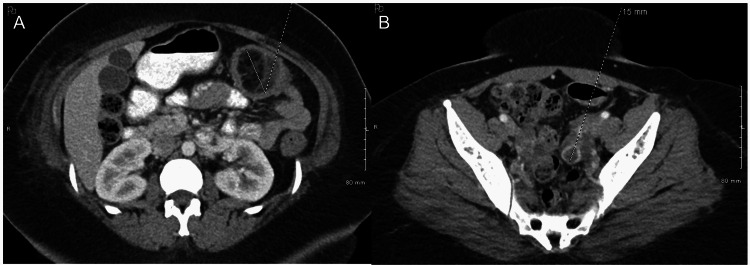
CT image revealed colo-colonic intussusception with lipomatous mass lesion (A, B); dotted lines in figure A are consistent with the target sign
